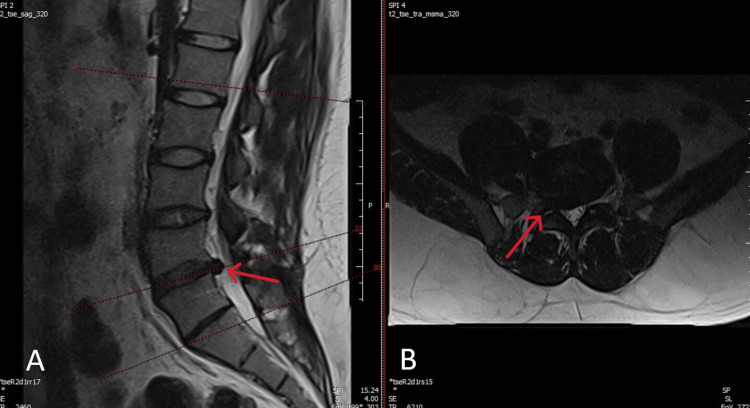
Preoperative lumbar MRI. (A) Sagittal T2-weighted MRI demonstrating L4–L5 disc extrusion (red arrow). (B) Axial T2-weighted MRI showing right paracentral disc herniation compressing the traversing right L5 nerve root (red arrow).
